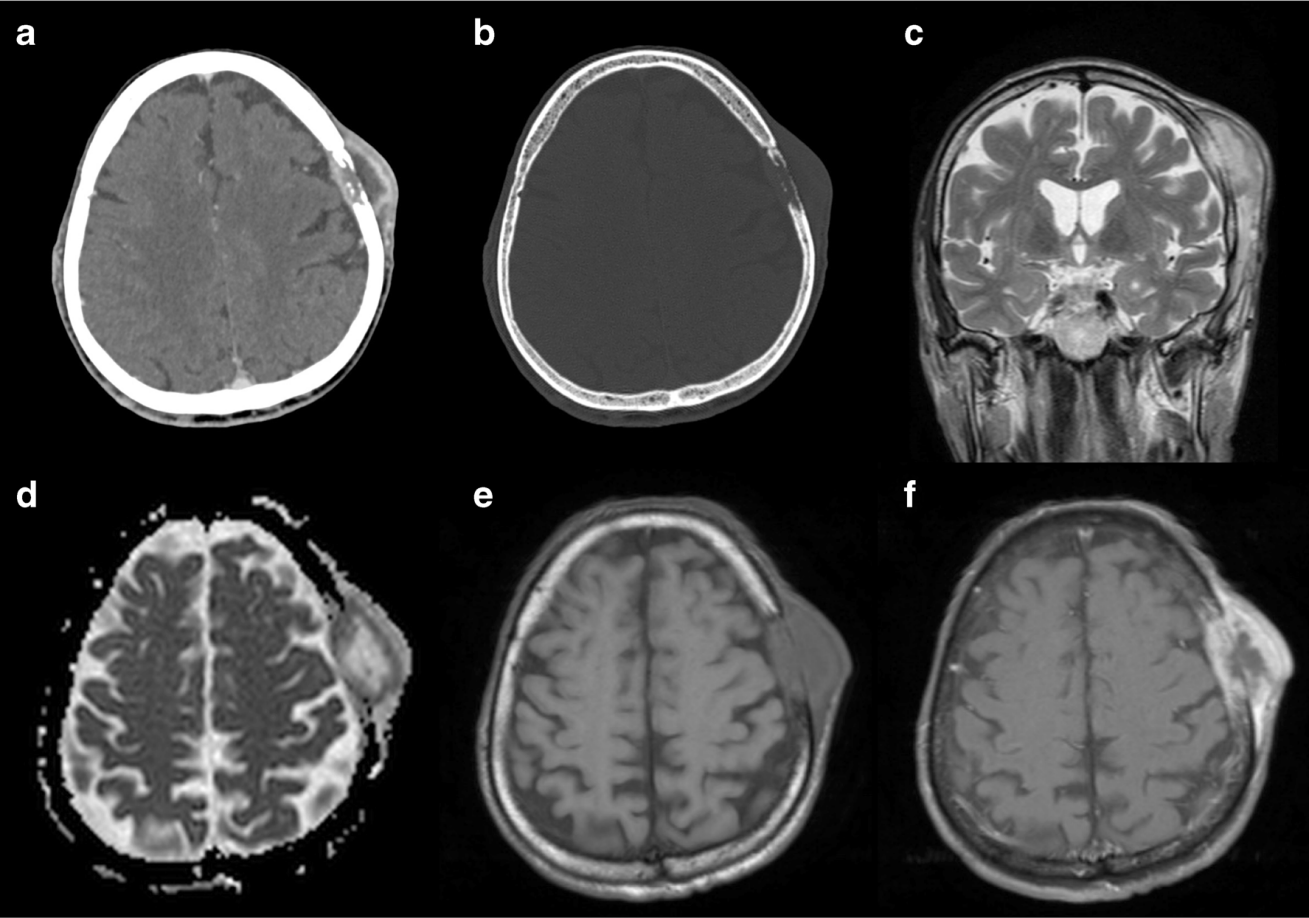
Metastasis. Axial contrast-enhanced CT, with brain (a) and bone (b) windows showing a left parietal lytic, widely necrotic, lesion with intra- and extracranial extension. MRI better depicts extraosseous spread on both intra- and extracranial aspects, with dural displacement and thickening, better seen on coronal T2w image (c). The lesion presents diffusion restriction (d) and enhancement (e, f) of the peripheral solid component. T1w (e) and T1w after contrast administration with fat saturation (f) images
